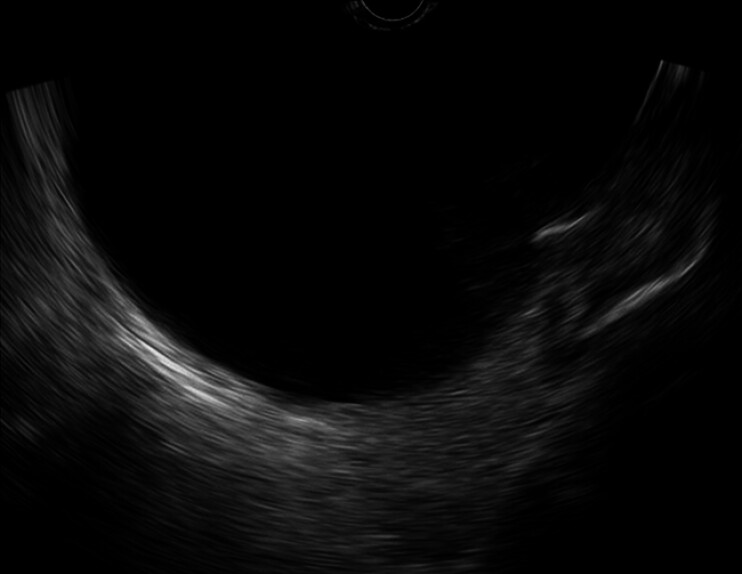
Endoscopic ultrasound image confirms a very large nonseptated, well-defined fluid collection.
